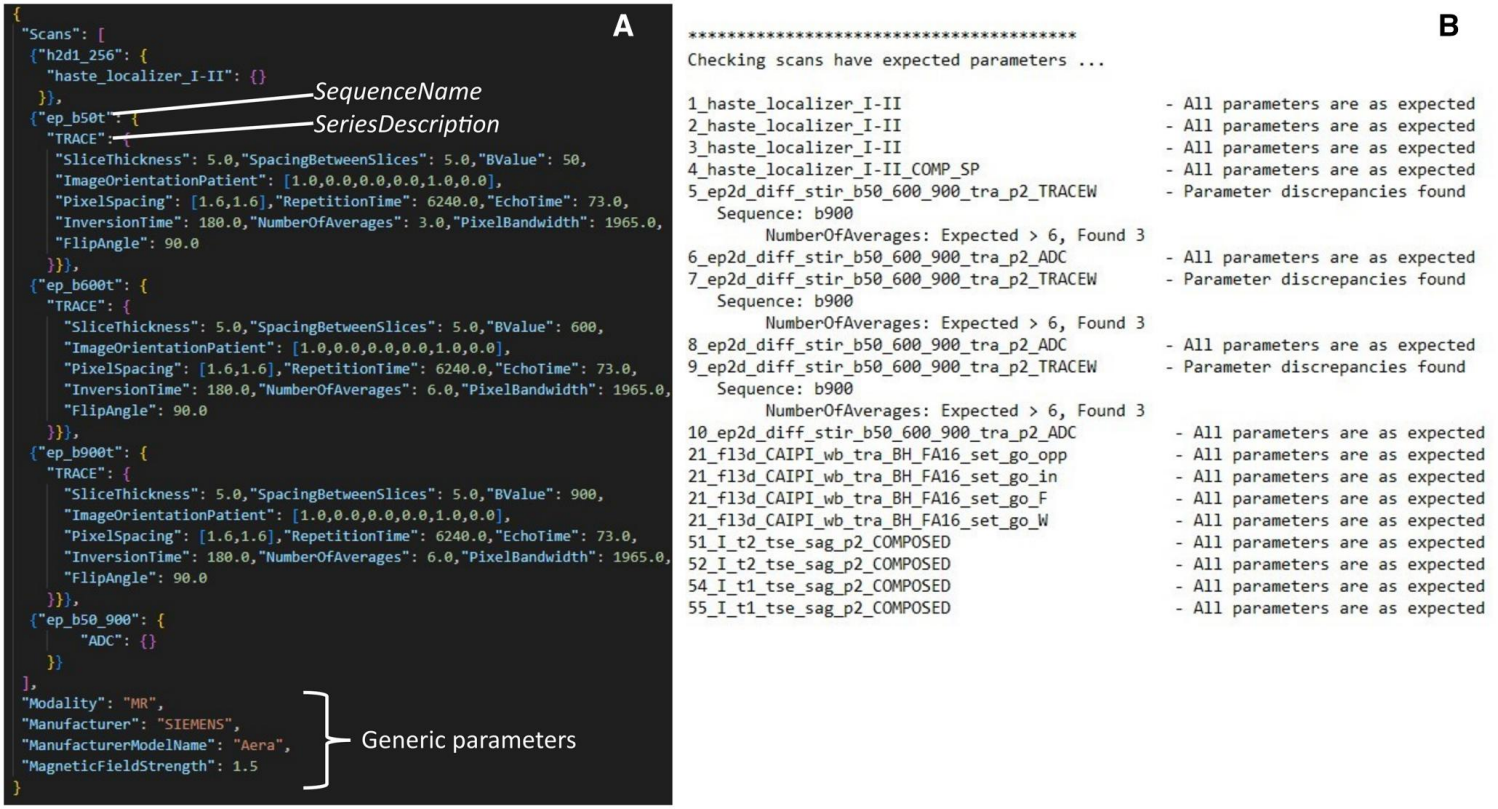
Example input and output files. (A) Extracts from the master file, defining the expected imaging protocol for a specific site. Series are identified using the *Sequence Name* and *Series Description* DICOM metadata tags and the values of each expected parameter are defined for b50, b600 and b900 DWI. The apparent diffusion coefficient (ADC) map is listed as an expected series but does not have any parameters defined. Generic parameters, which are expected to be the same for all series, are listed at the bottom of the file. (B) An extract from the results file produced by Protocol Checker, highlighting parameter discrepancies for DW and Dixon imaging.
